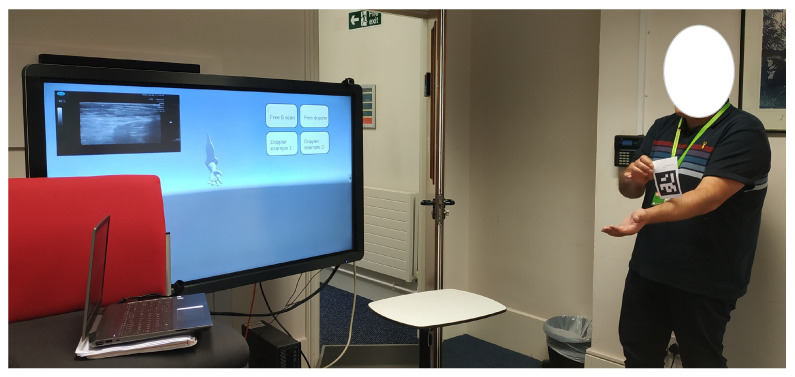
Participant testing the ultrasound training system.
